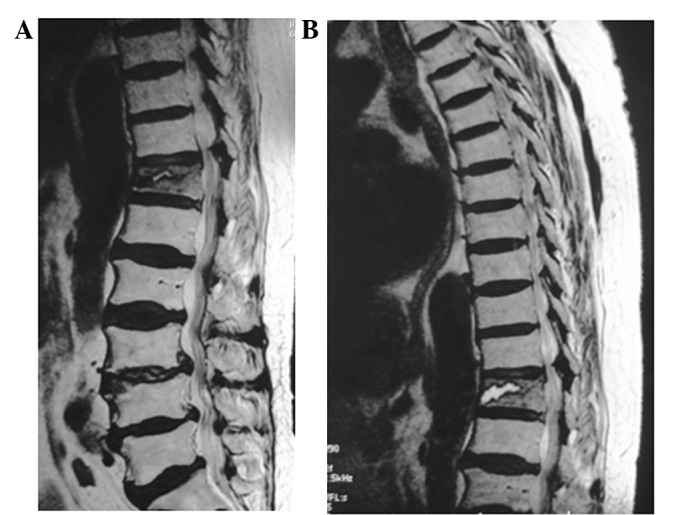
MRI scans for a 70-year-old female patient suffering from Kümmell’s disease at T12. (A) The lumbar MRI, T2-weighted image showed a mixed signal of gas and liquid at T12 and (B) 8 min later, the thoracic MRI, T2-weighted image showed a hyperintense signal of liquid at T12.
